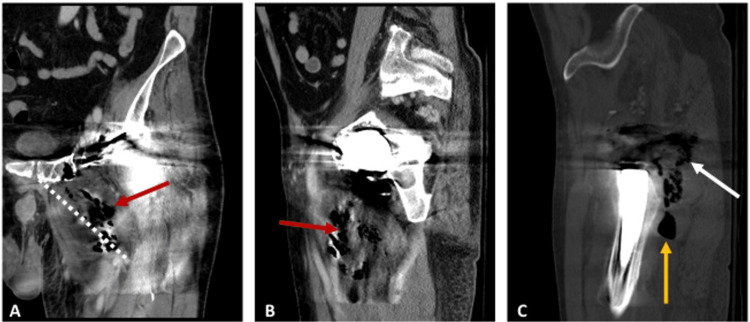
Original left hip computed tomography scan from the outside hospital (A) Coronal view of the largest abscess collection (red arrow), measuring 8.3 x 3.7 x 6.7 cm and tracking from the symphysis pubis to the middle thigh (dashed-white line). (B) The aforementioned abscess is viewed from a sagittal image. (C) Severe cellulitis and diffuse myofascitis (white arrow) and additional abscess (gold arrow) in the buttock and posterior thigh.
